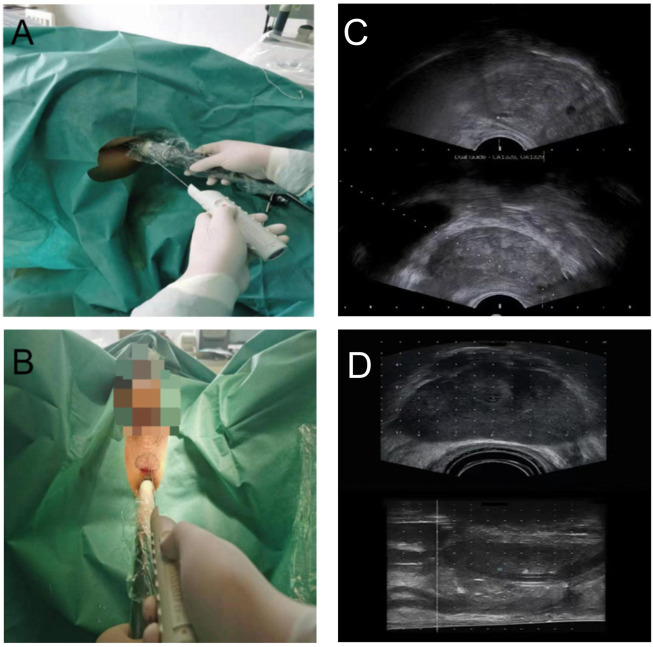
(A) Transrectal personalized prostate biopsy. (B) Transperineal personalized prostate biopsy. (C) Ultrasound images of transrectal biopsy. (D) Ultrasound images of transperineal biopsy.
